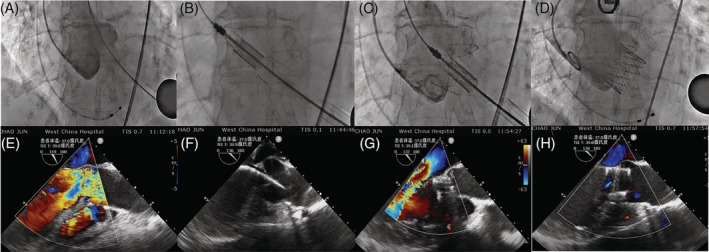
Intraoperative angiogram and TEE images of TAVR procedure by J‐Valve system with pure aortic regurgitation. A, Aortic root angiogram to set up optimal C‐arm angulation. B, Prosthesis was inserted above aortic annulus to the ascending aorta and locators were deployed. C, Prosthesis descended to the annulus level and aortic root angiogram to verify the position of prosthesis and locators. D, Successful prosthesis release and postoperative aortic root angiogram again. TEE during the procedure: E, TEE of native aortic valve severe regurgitation before THV implanted. F, TEE of prosthesis was inserted above aortic annulus; G, TEE prosthesis descended to the annulus level; H, TEE of aortic stent valve well positioned after THV implanted. TEE, transesophageal echocardiography; THV, transcatheter heart valve
